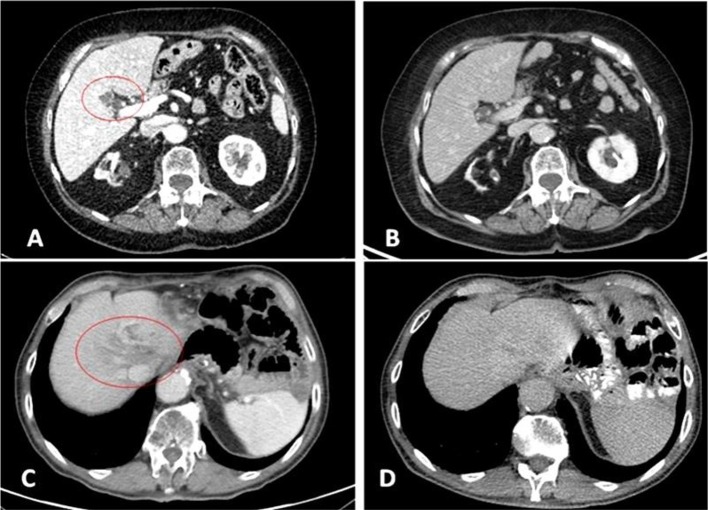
2017 pre-treatment (A = patient 1; C = patient 2) and 2019 complete response CT scans (B = patient 1; D = patient 2). Liver metastases at IV (A) and VIII (C) segment.
